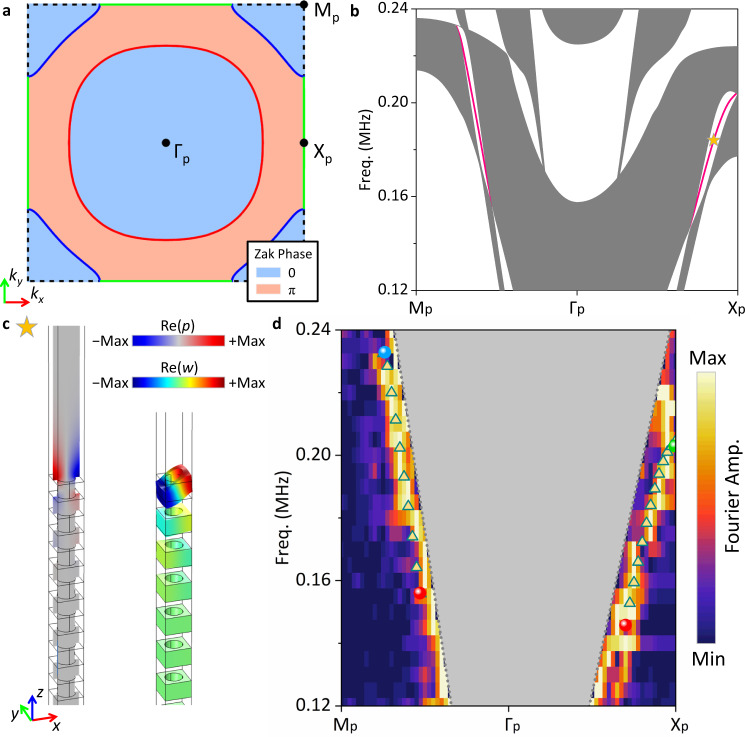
Strongly tilted drumhead surface state (DSS) between nodal rings. a Distribution of Zak phase on the surface FBZ projected along *z* direction. b Calculated projected band structure of a supercell terminated by *x*-*y* surface. Solid lines represent the strongly tilted DSS. Gray shaded regions denote the projected bulk bands. c Calculated field maps of acoustic pressure (Re(*p*)) and elastic displacements (Re(*u*), Re(*v*), Re(*w*)) for the marked point in (b). The thin solid lines outline profile of the supercell without elastic displacements. d Experimental Fourier spectra along high-symmetry directions when exciting and detecting the ultrasound at the same side of the phononic crystal. Triangle scatters: the calculated dispersion of the strongly tilted DSS along the high-symmetry directions.
